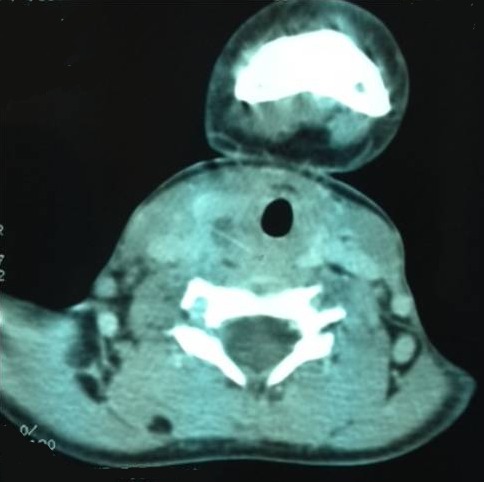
CT scan axial view that shows a right retro-thyroidien abscess and a foreign body (thorn: black arrow)
